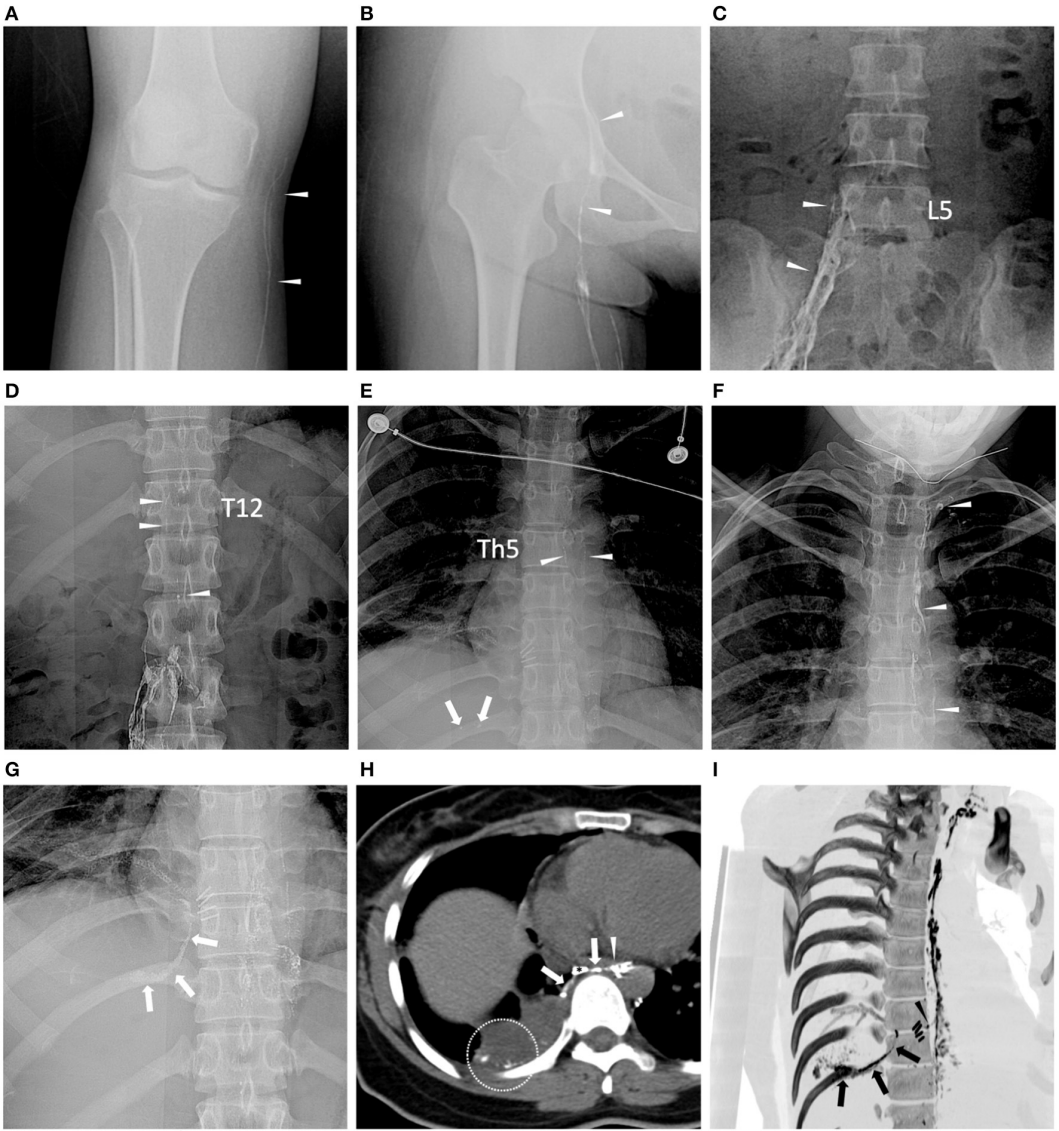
Dynamic opacification of lymphatic vessels in fluoroscopy and chest CT. Images were obtained from the same patient with non-small cell lung carcinoma (*Patient ID: 3*). (A–F) 3, 9, 22, 45, 52, and 60 min after ethiodized oil injection, respectively, lymphatic vessels (white arrowheads) at the level of knee joint space, upper femoral head, L5, Th12 and Th5 vertebrae, and left jugular venous angle were consecutively opacified and dotted ethiodized oil extravasation [(E) white arrows) can be observed in the course. (G) After completing the ethiodized oil injection (28.0 ml), fluoroscopy demonstrated more definite ethiodized oil extravasation (white arrows). (H) Following CT (axial image with 5 mm thickness) after TL showed a rupture of the thoracic duct (white arrowhead) near the metal clips (*) and thread-like ethiodized oil extravasation (white arrows) can be observed, as well as the free ethiodized oil in the right pleural effusion (within the white circle). (I) Reconstructed oblique CT image with maximum Intensity projection (MIP) showed more intuitive opacification of the thoracic duct, leakage site (black arrowhead) near the metal clips, and the ethiodized oil extravasation in the right pleural cavity (black arrows). It indicated a possibility of iatrogenic damage to the thoracic duct in the surgery.
